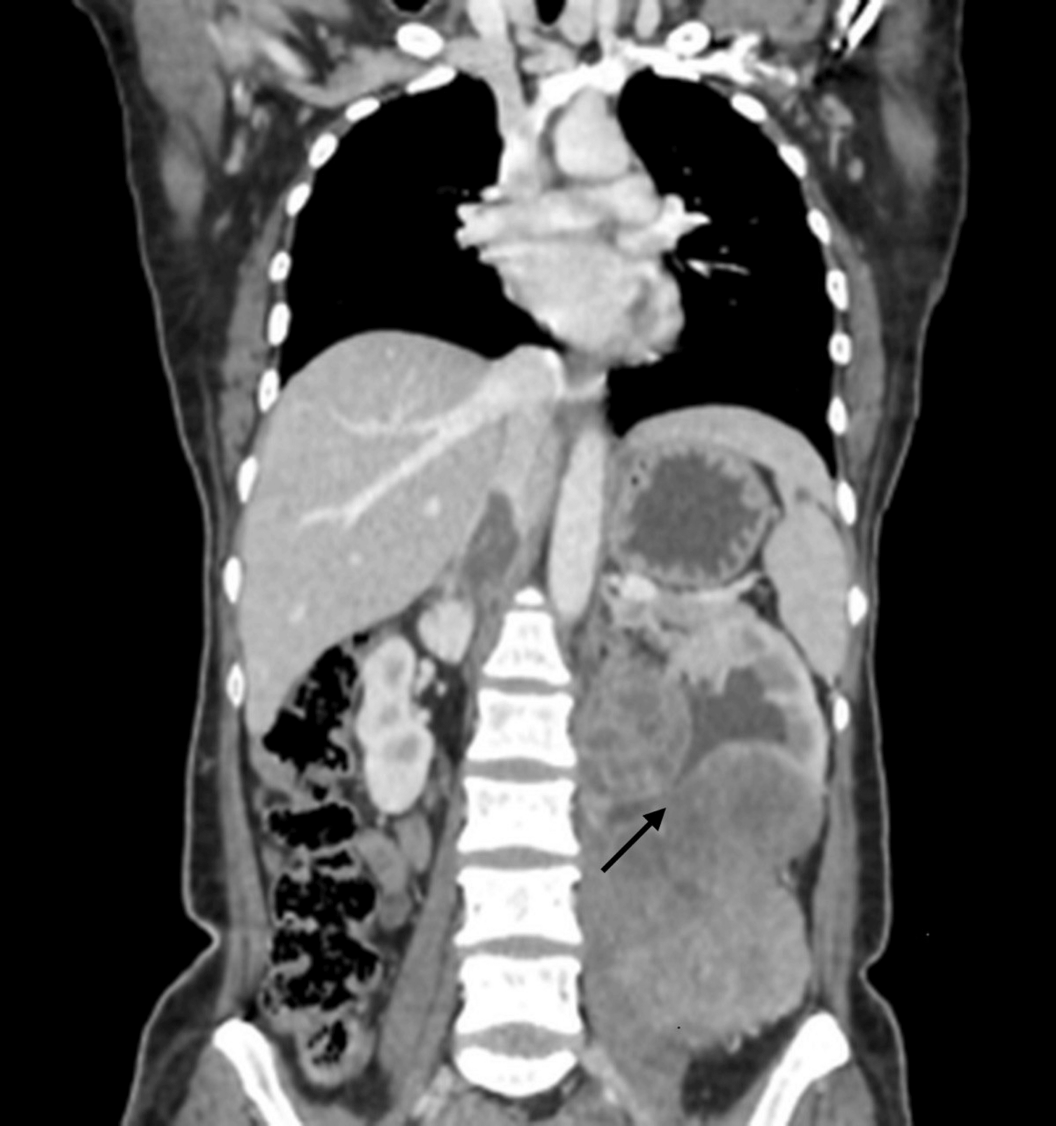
CECT abdomen coronal section showing encasement of the left proximal ureter by the lesion (arrow), causing moderate hydroureteronephrosis. CECT: contrast-enhanced computed tomography
